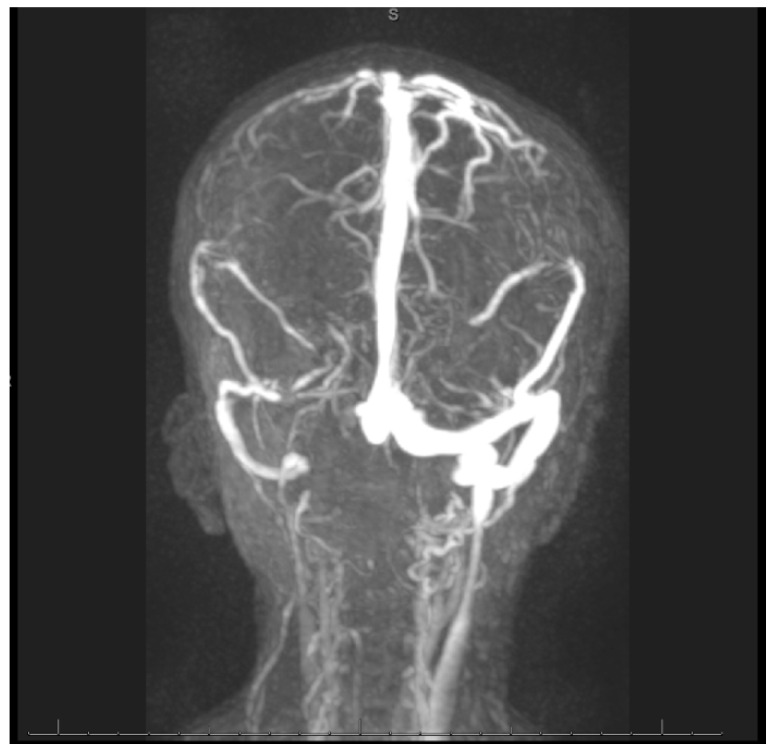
Brain and neck computed tomography with contrast.
